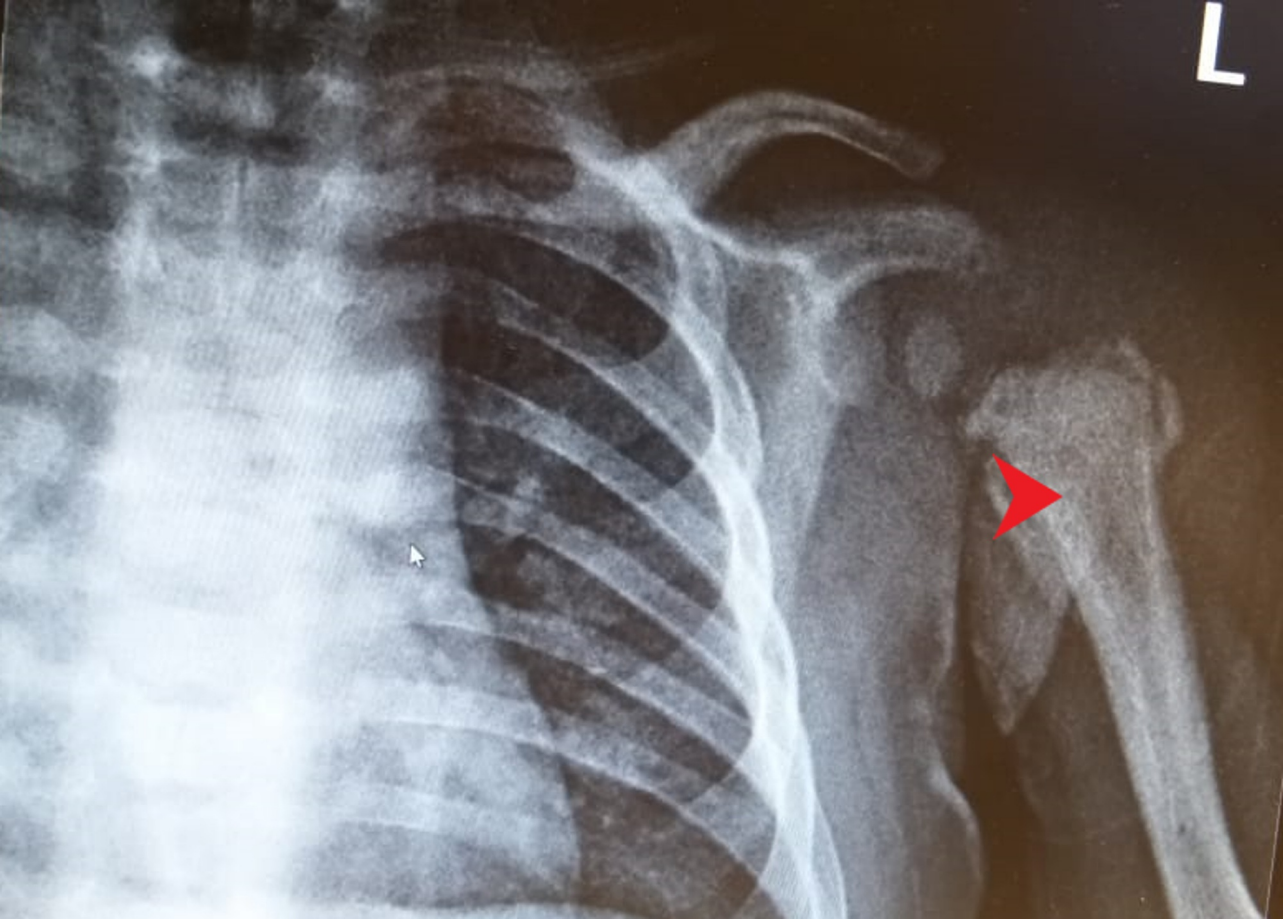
X-ray of the left shoulder shows periosteal reaction with new bone formation.
